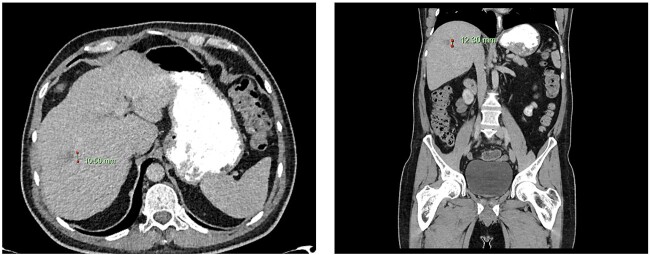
Computed tomography dated 15 January 2013 of the abdomen and pelvis measuring 10.50 mm (anterior–posterior) lesion at liver segment 5/6 in axial (left) and coronal (right) view.
